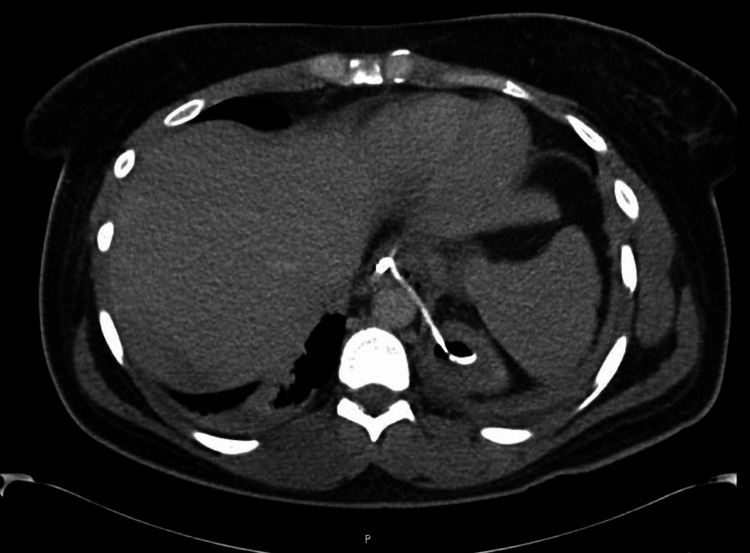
CT of the abdomen/pelvis with contrast, transverse view, showing the stent in place.
